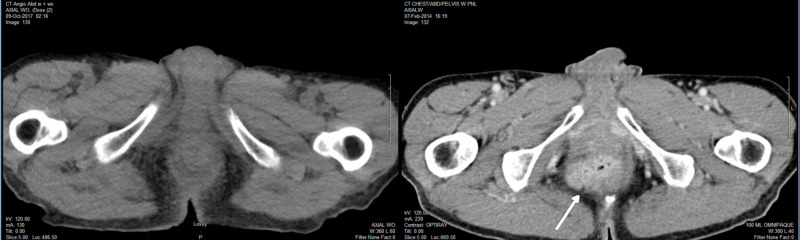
Last follow-up CT scan showing no local recurrence on the left, versus the large tumor (white arrow) on the pre-treatment CT on the right CT, computed tomography
